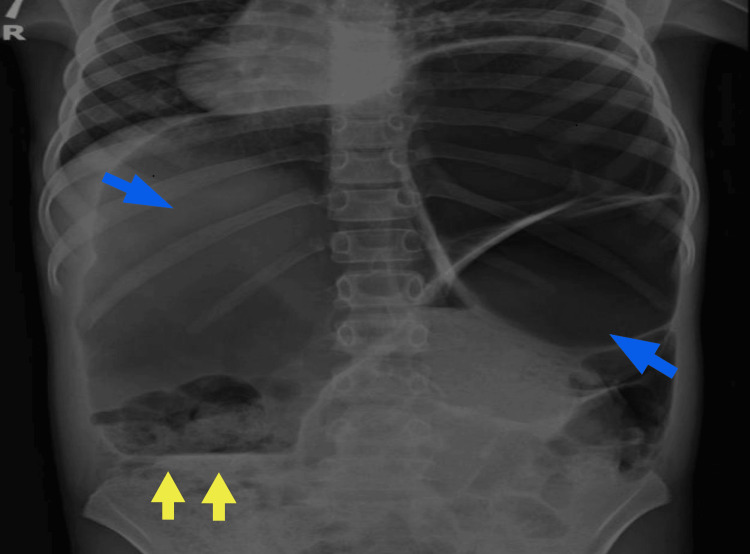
Abdominal radiograph demonstrating dilated large-bowel loops with peripheral air-fluid levels (yellow arrows) and the classic “coffee-bean” sign (blue arrows).
